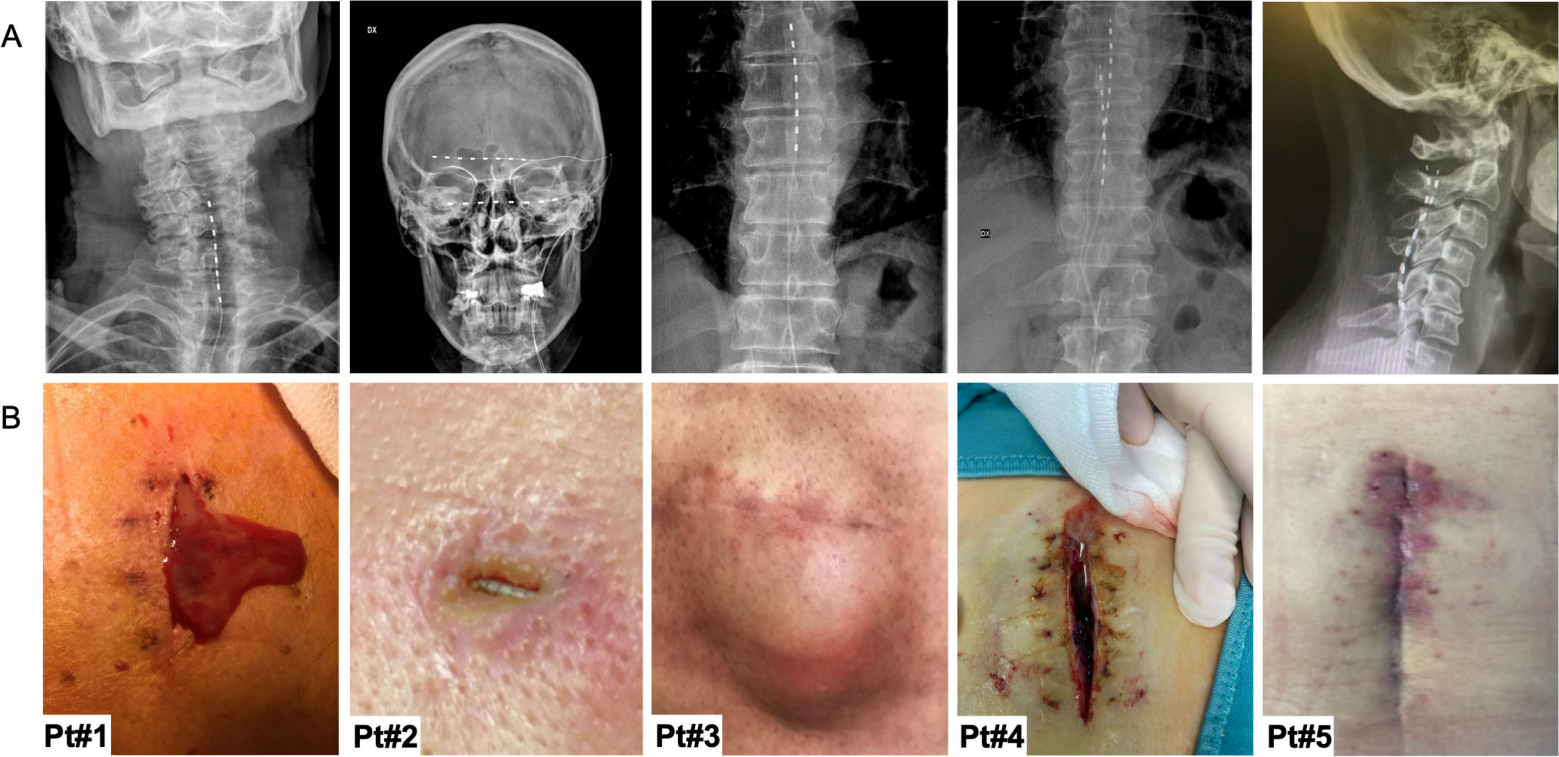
(A) Representative radiographic images of different implantable neuromodulators, and (B) associated tissue infections for five patients (Pt).
